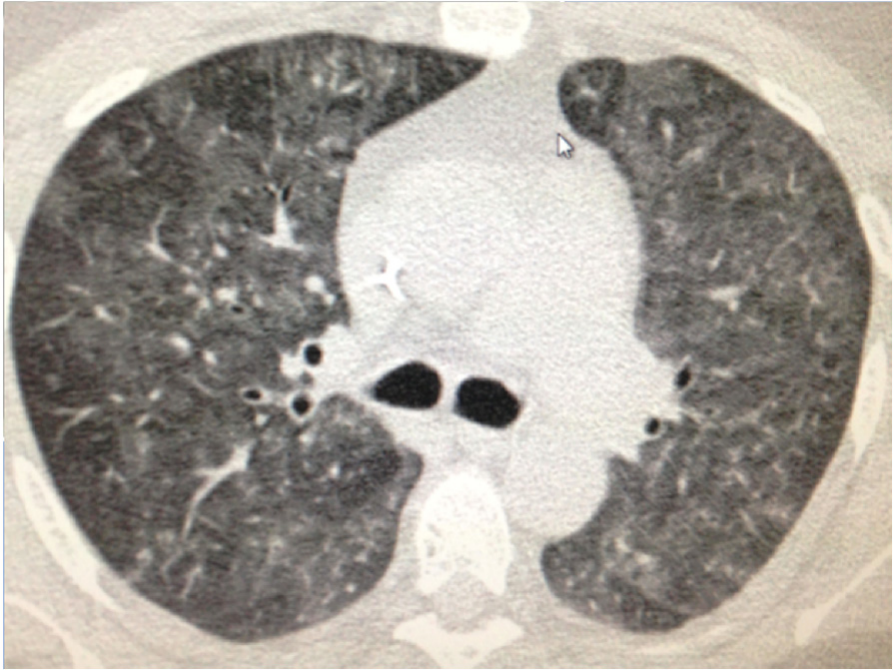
A 24-year-old man on chemotherapy for non-Hodgkin lymphoma presenting with thrombocytopenia and hemoptysis. Axial unenhanced CT image of the thorax showing diffuse bilateral ground-glass opacities, compatible with alveolar hemorrhage.
